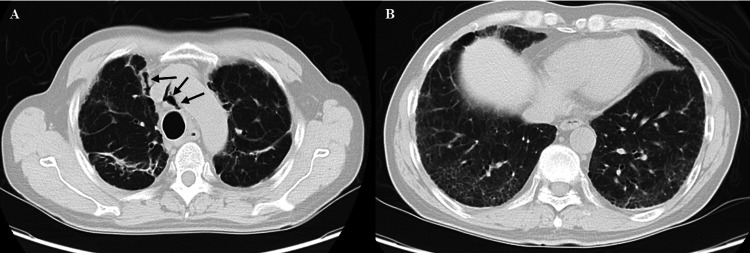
Follow-up chest CT after corticosteroid therapy Significant improvement of ground-glass opacities is observed in both lungs following steroid treatment. However, reticular opacities remain, and new-onset pneumomediastinum is identified (black arrows).
